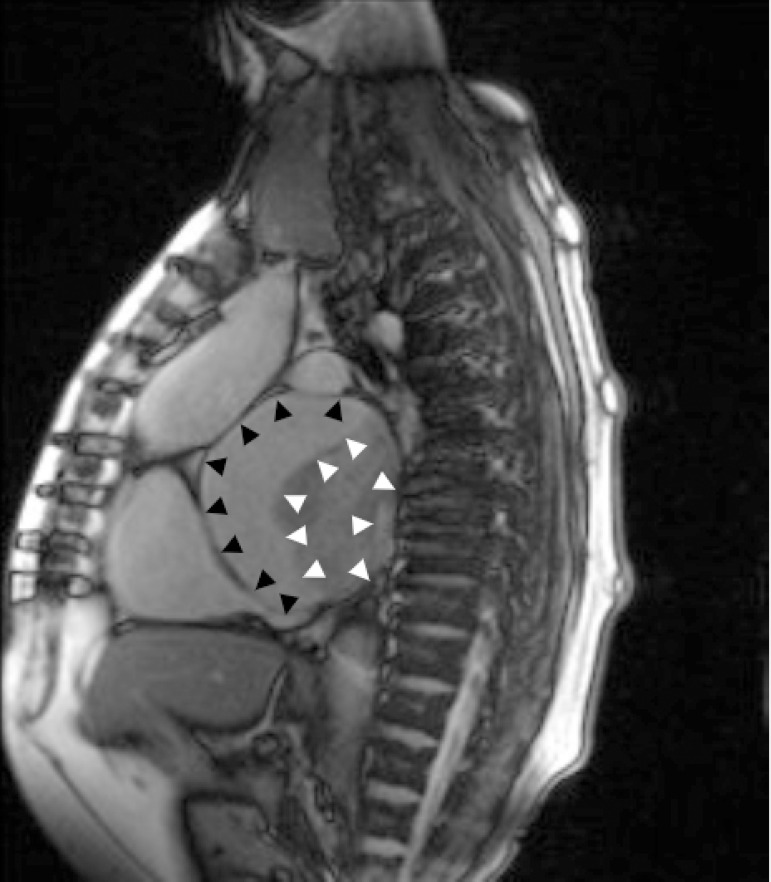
Parasagittal magnetic resonance imaging of the thorax. We observed a giant left atrium (dark arrows) occupied by irregular smooth mass (white arrows).
